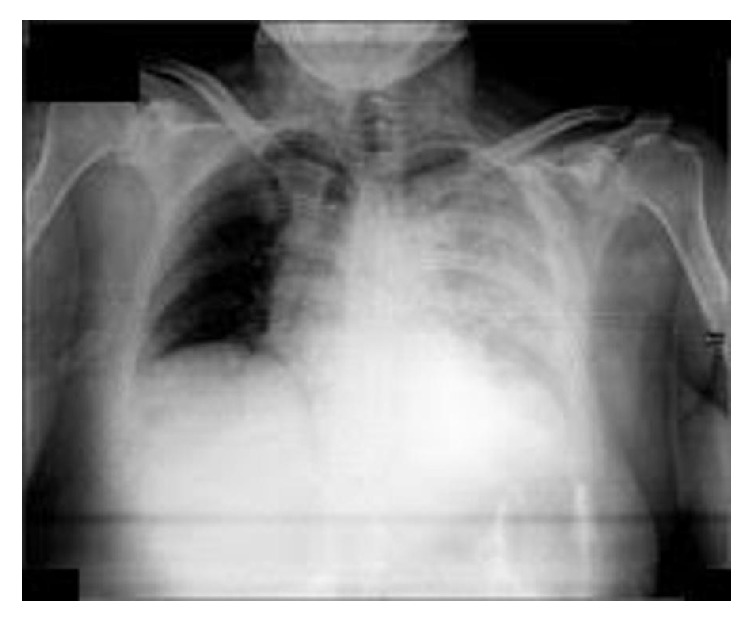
Chest X-ray (three hours postoperatively) demonstrating left pleural effusion.
